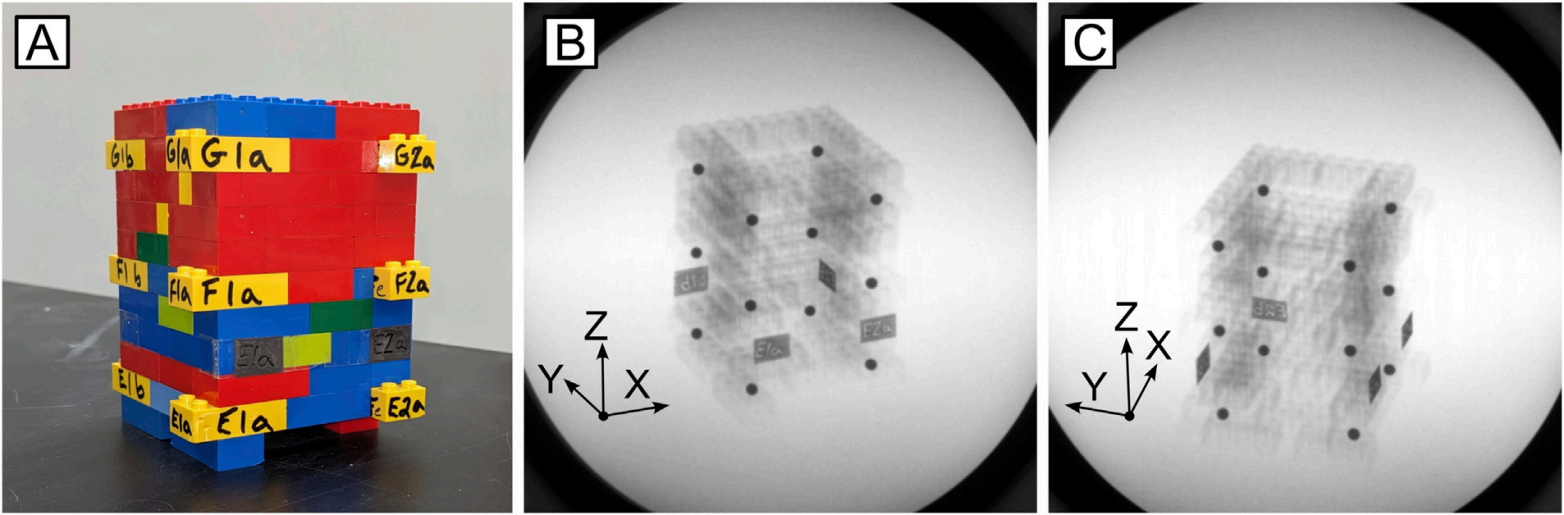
Calibration cube utilized to calculate RMS error associated with the mechanical testing system-induced magnetic field. (A) Image of the LEGO cube; (B) Inline and (C) offset x-rays of known bead locations.
